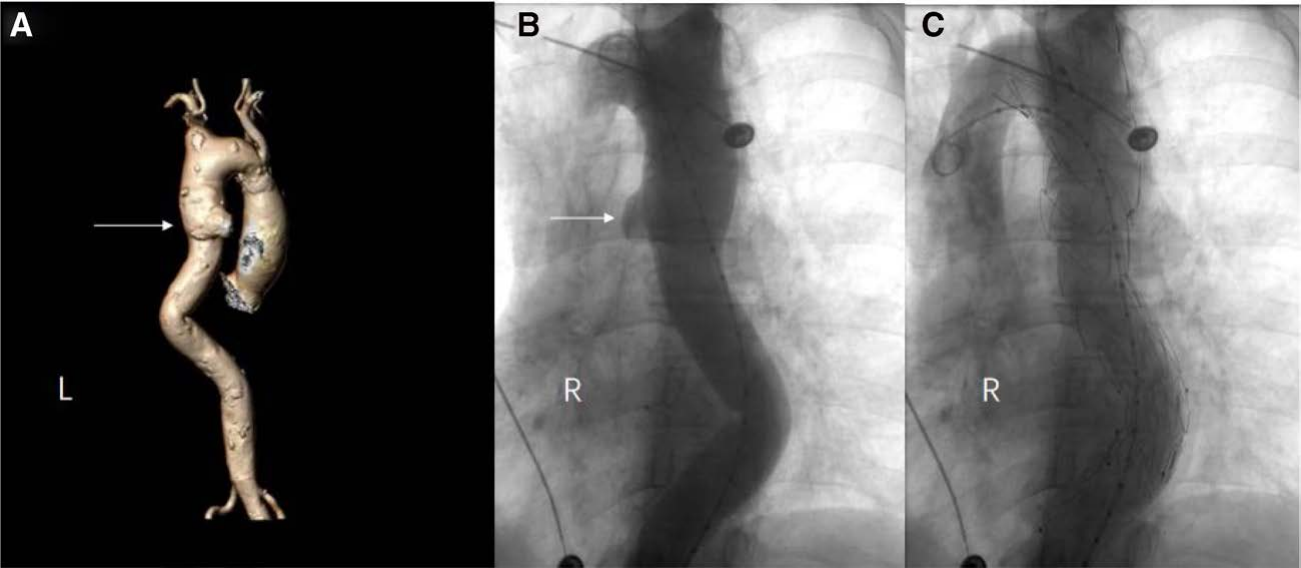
(A) CTA of the thoracoabdominal aorta shows a penetrating ulcer in thoracic aorta. (B) Intraoperative DSA shows an aortic ulcer approximately 2 cm distal to the left subclavian artery on the lesser curvature of the aorta. (C) Intraoperative DSA shows no endoleaks. CTA = computed tomography angiography, DSA = digital subtraction angiography.
